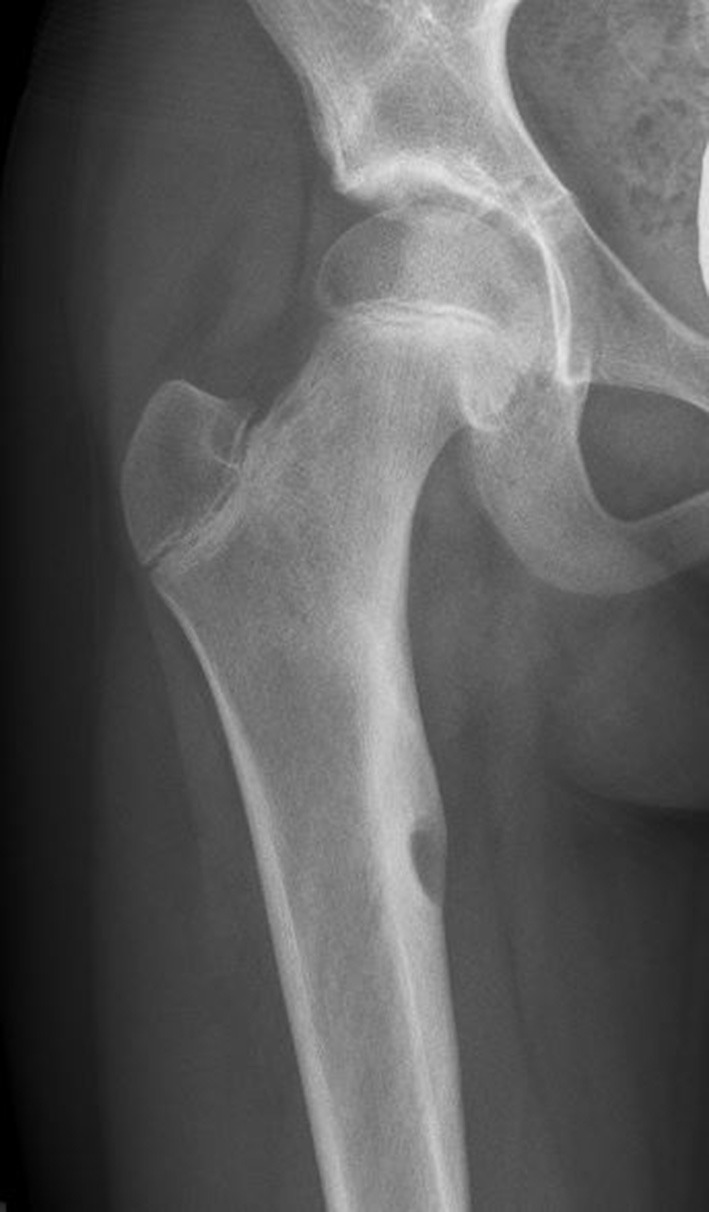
Involvement of the proximal right femur. Anterior-posterior radiograph shows a well-defined lucent lesion in the proximal diaphysis of the right femur with surrounding sclerosis, indicating chronic osseous destruction.
